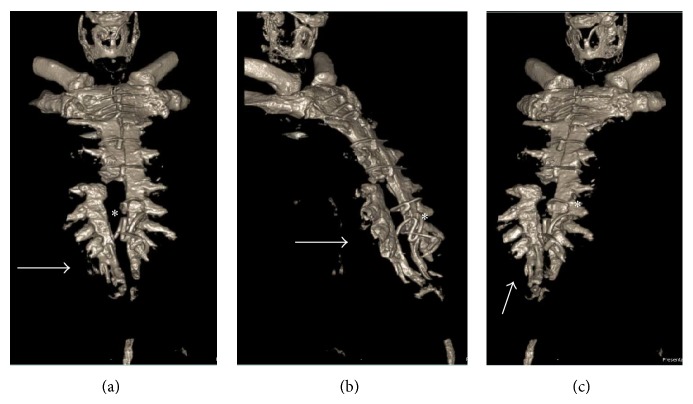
Preoperative 3-dimensional computed tomography showing the right lower part of the “floating” sternum. (a) Anterior-posterior view, (b) right anterior-oblique view, and (c) left anterior-oblique view showing the dehisced right lower part of sternum (highlighted with an* arrow*) with sternal wires that have pulled through (highlighted with an* asterisk*).
